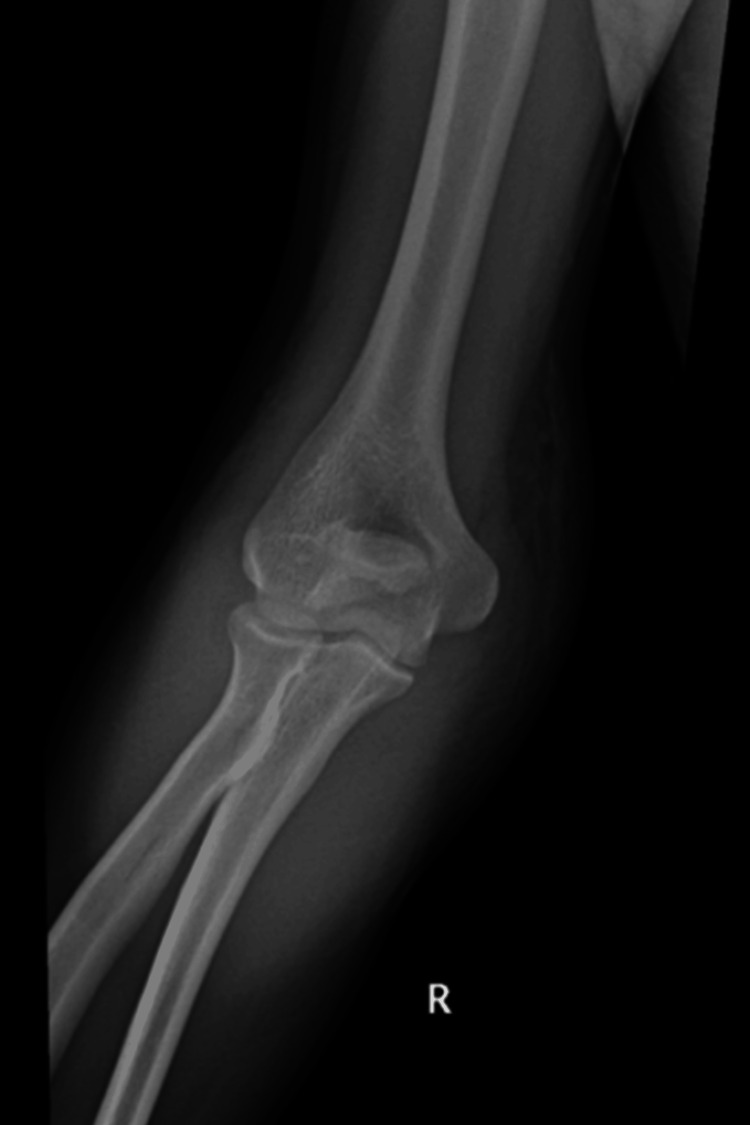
Preoperative AP X-ray image of the right elbow. AP, anteroposterior
